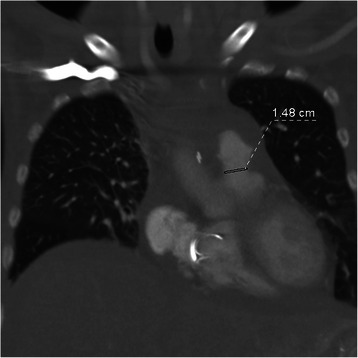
Computed tomography of the thorax with stenosis of the distal anastomosis of the pulmonary valve conduit with a diameter of 14.8 mm.
